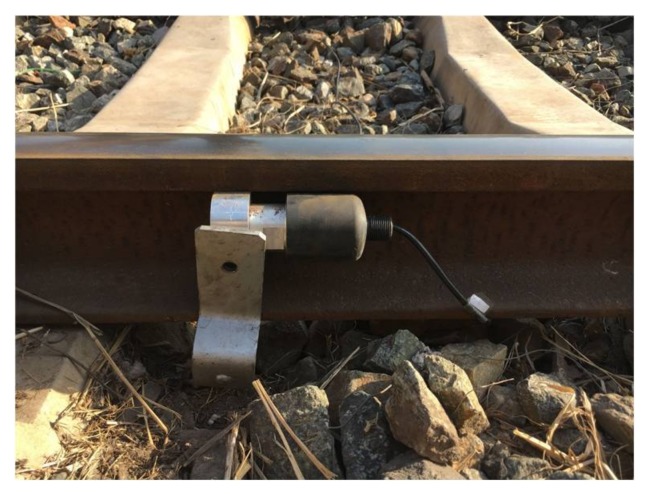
Installation of ultrasonic transducer at rails.
